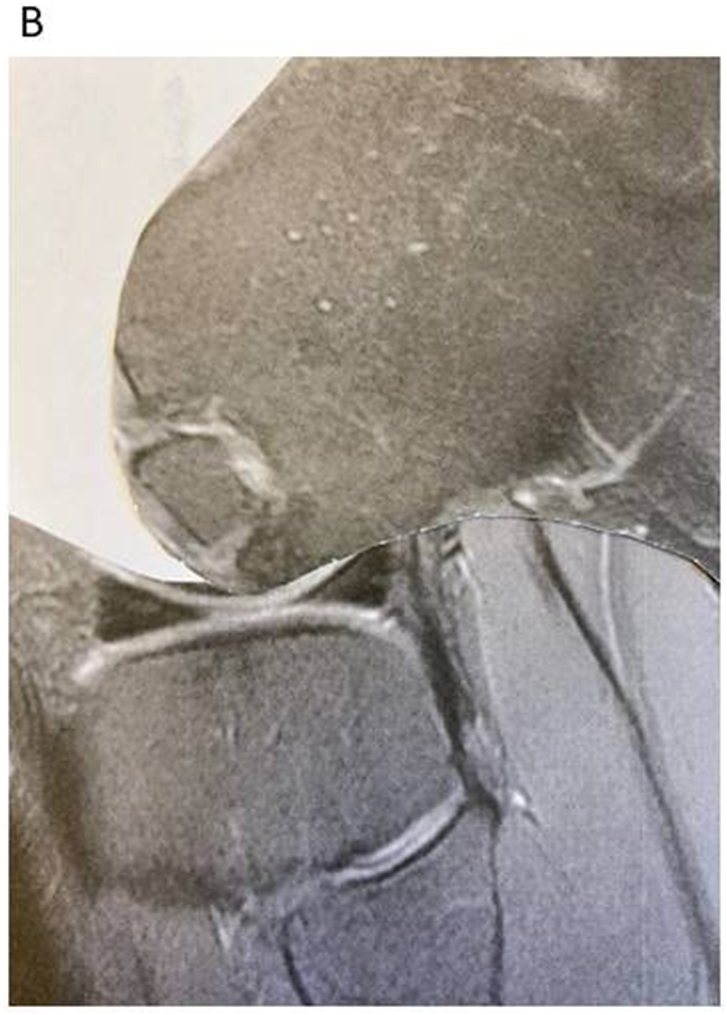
By printing out the sagittal MRI image and cut the image along the femoral condyle lining, one may rotate the cut area to make the image like a maximal flexion. By such a simple procedure, it is possible to get an idea if it is possible to use a trans arthroscopic intervention; the ¨Cut doll-test¨.
